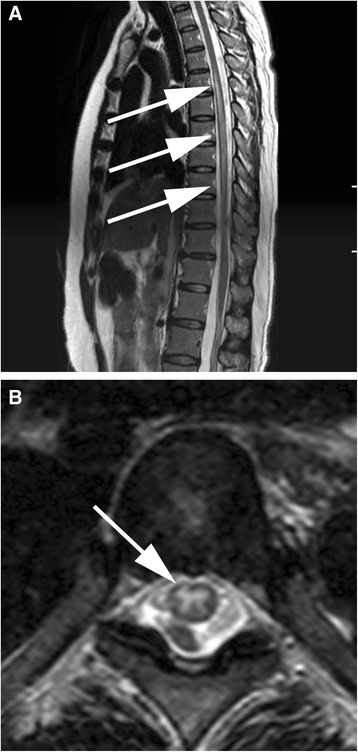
Intramedullary hyperintensity, particularly in the gray matter, extending from T5–T9 levels on T2-weighted magnetic resonance imaging (*white arrows*). a Sagittal view. b Axial view
